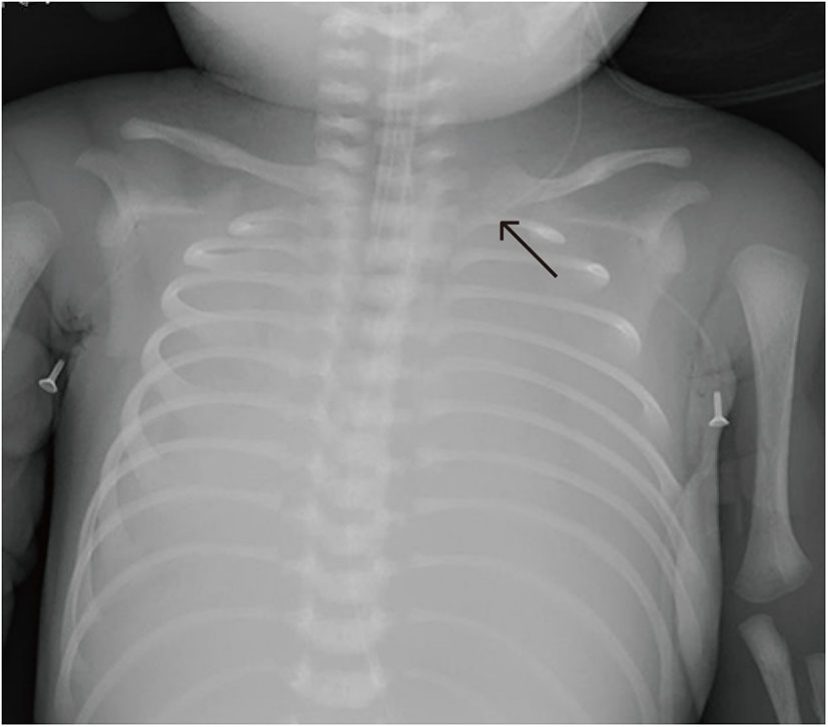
Patient C was transferred from a local hospital because of acute respiratory distress. Continuous parenteral nutrition infusion via a non-central Epicutaneo cava catheter (ECC) resulted in PE, with the chest X-ray showing bilateral “white lung”.
